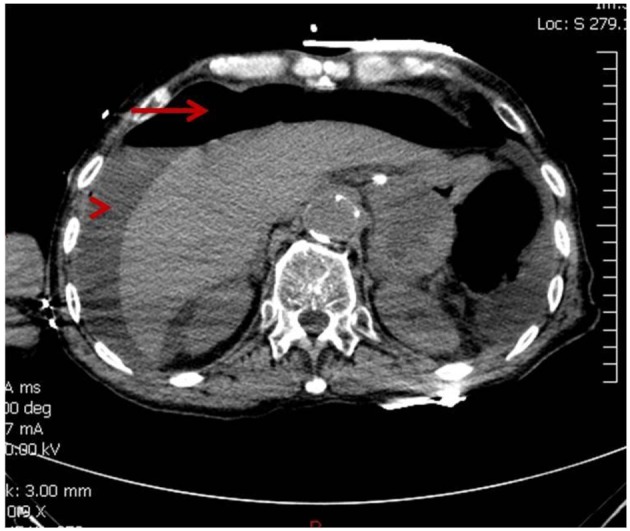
CT showing moderate pneumoperitoneum (arrow) and perihepatic fluid (arrow head).
